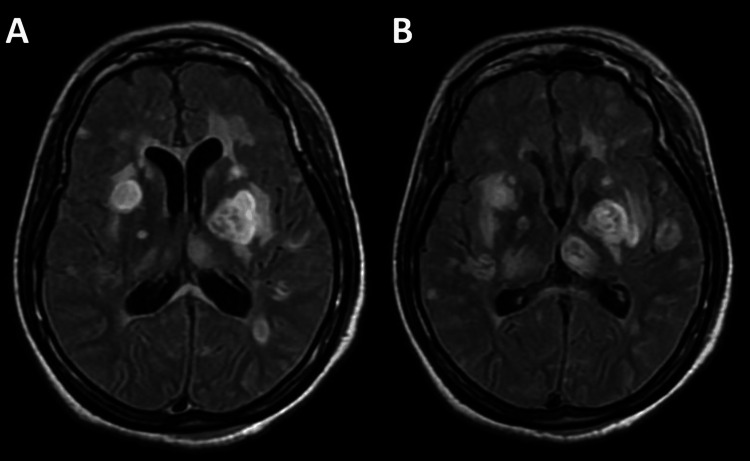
Brain MRI, axial T2/FLAIR images MRI: magnetic resonance imaging; FLAIR: fluid-attenuated inversion recovery; HIV: human immunodeficiency virus (A) Hyperintense lesion involving the basal ganglia with surrounding vasogenic edema. (B) Additional multifocal hyperintense lesions in the deep white matter, consistent with cerebral toxoplasmosis in the setting of advanced HIV infection
